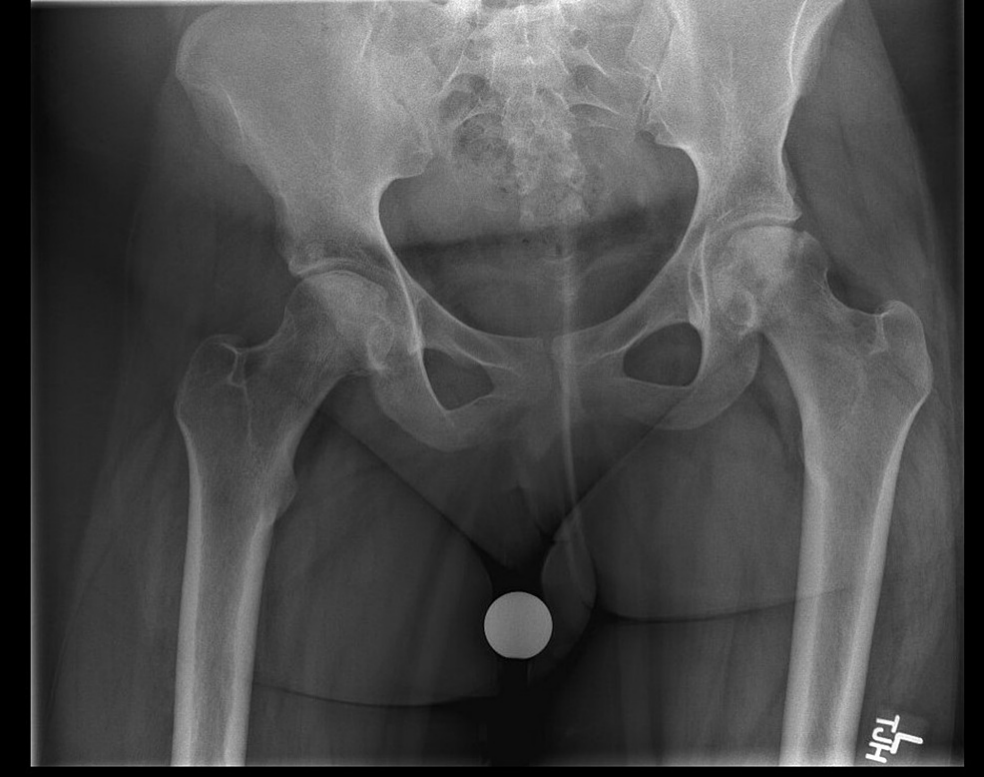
X-ray of the pelvis Single frontal view of the low pelvis demonstrating bilateral femoral head avascular necrosis. Mild subchondral collapse on the left. No definite subchondral collapse on the right.
